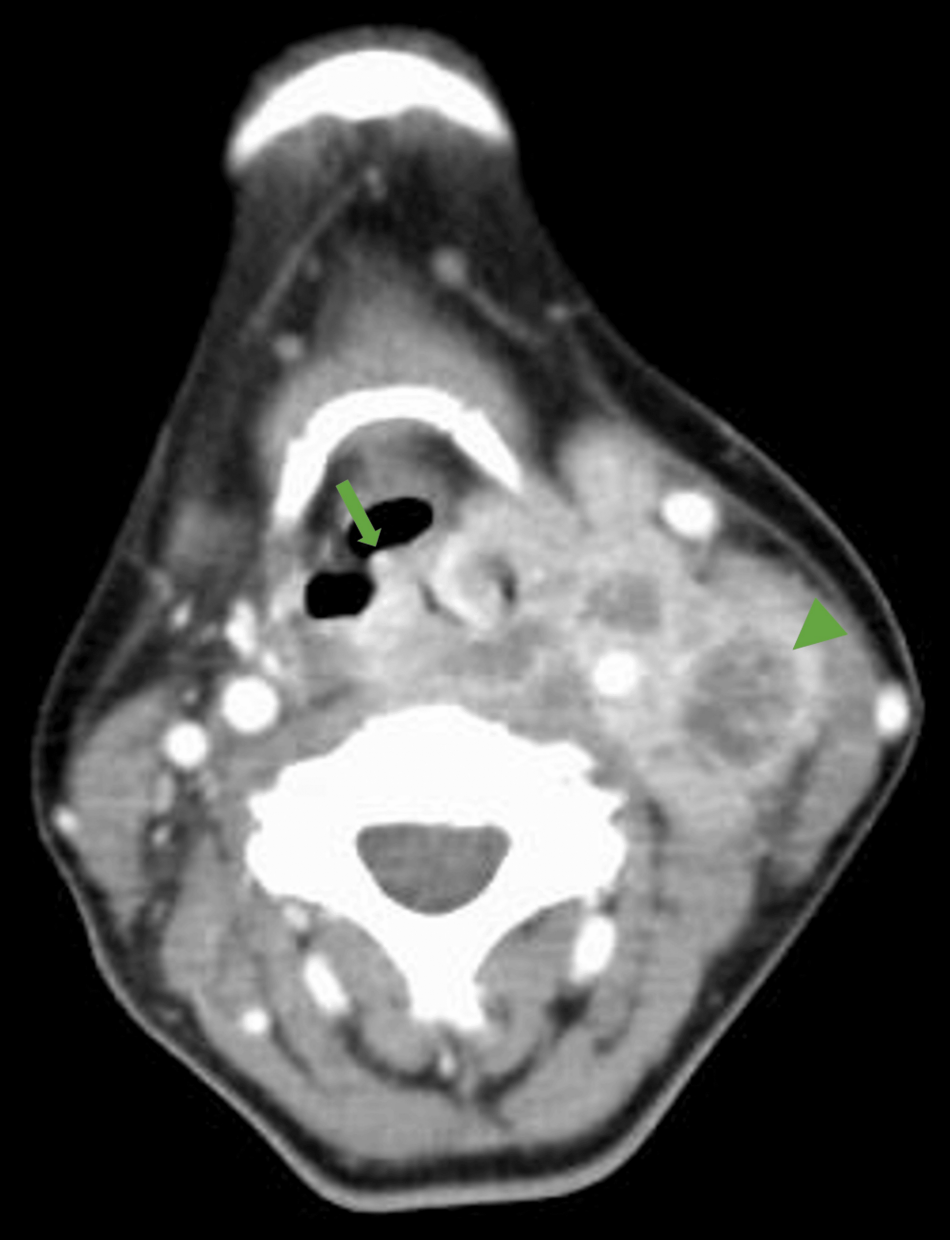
Contrast-enhanced computed tomography of the neck Contrast-enhanced computed tomography showed a thick pharyngeal wall (arrow) and lymphadenopathy with irregular nodular borders and central necrosis (arrowhead) at the level of the pre-epiglottic space.
